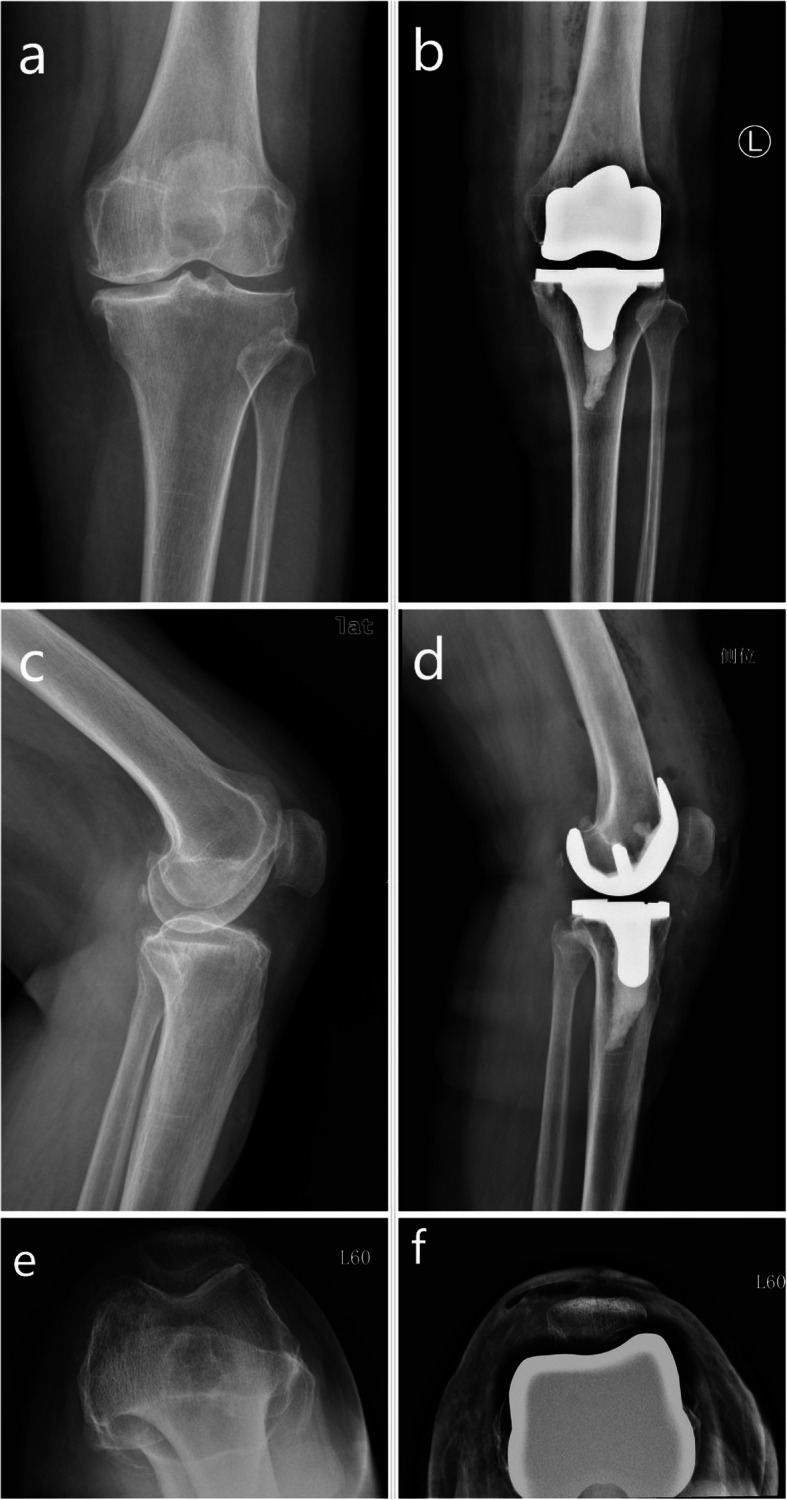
Typical case of medial pivot prosthesis. a Anteroposterior position before surgery in patients with medial pivot (MP) prosthesis. b Anteroposterior position after surgery in patients with MP prosthesis. c Lateral position of the knee joint before surgery in patients with MP prosthesis. d Lateral position of the knee joint after surgery in patients with MP prosthesis. e Patella axial radiograph (60°) before surgery in patients with MP prosthesis. f Patella axial radiograph (60°) after surgery in patients with MP prosthesis
